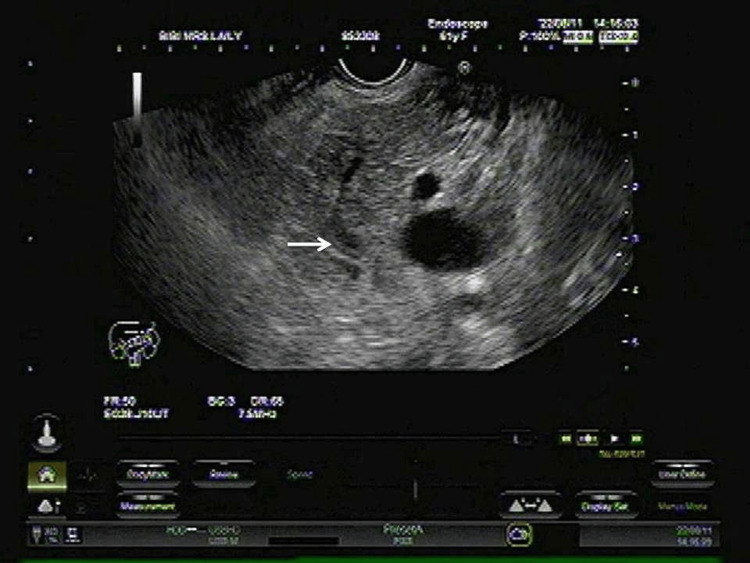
Linear endoscopic ultrasound showing a long, linear, hyper-echogenic structure without acoustic shadowing in the main pancreatic duct (white arrow).
